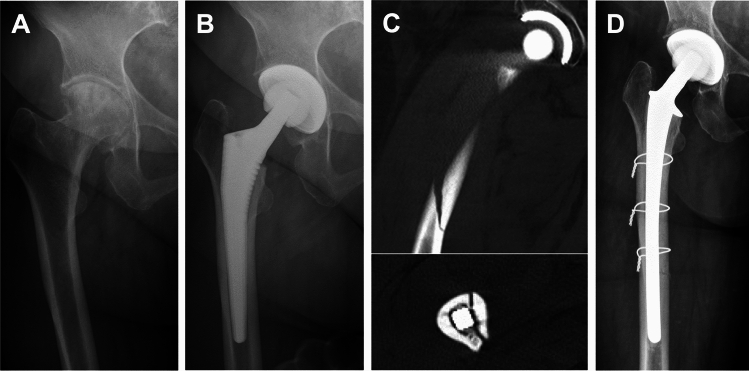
Exemplary case study of a 65-year-old woman with osteoporosis and a postoperative periprosthetic femoral hip fracture (PPF). A Preoperative radiograph showing end-stage OA and Dorr C type femur. B Postoperative radiograph after uncemented THA without evidence of a fracture, C computed tomography (coronal and axial view) showing a PPF (Vancouver type B2), D radiograph after stem revision
